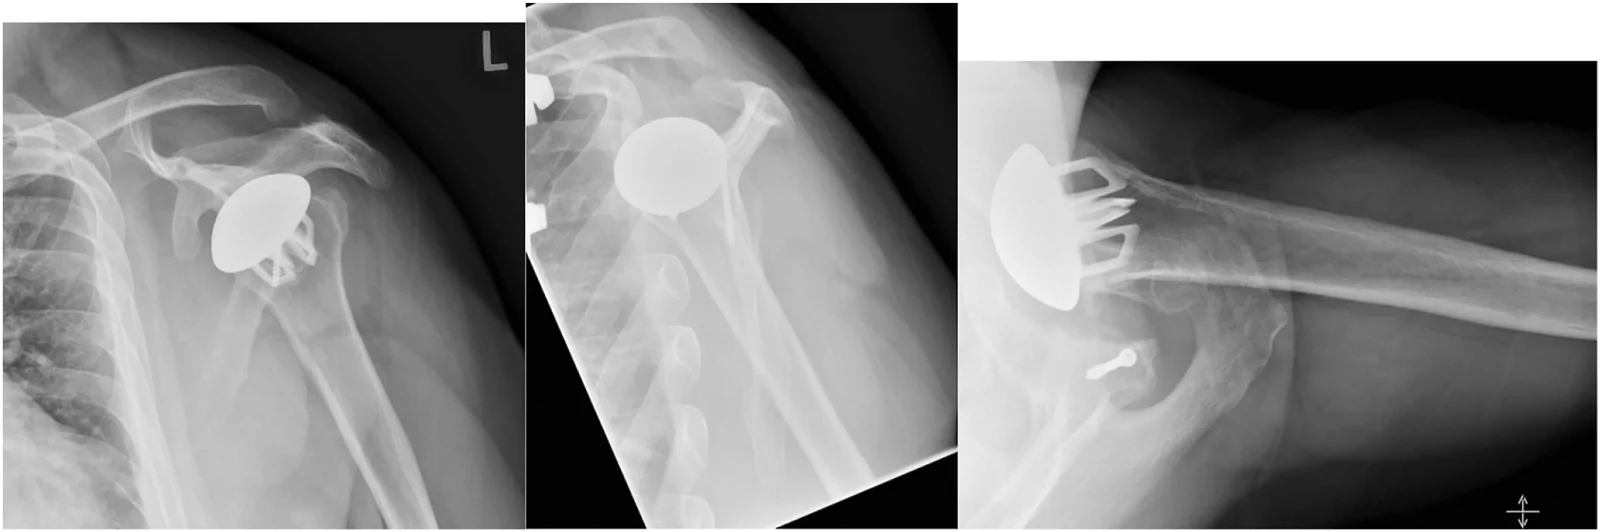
Case 2 pre-operative x-rays showing anterosuperior escape of a previous shoulder hemiarthroplasty.
